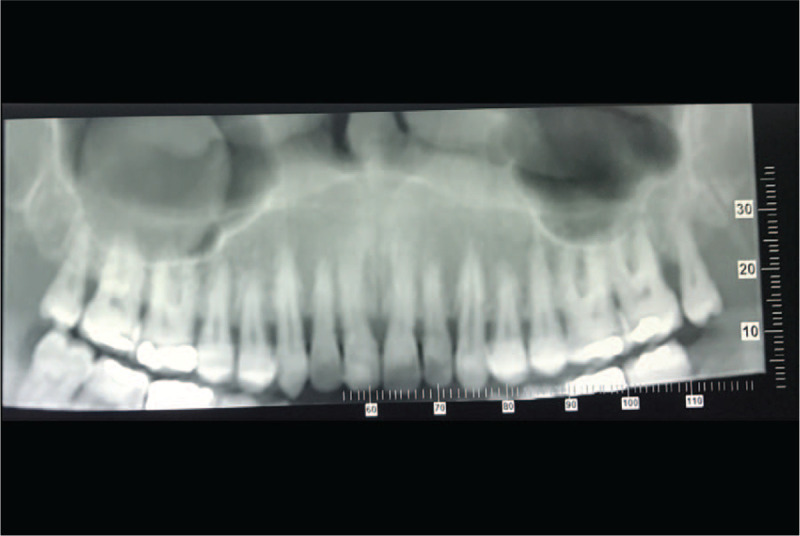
A dental panoramic radiography revealed a lesion of the upper left dental arch.
